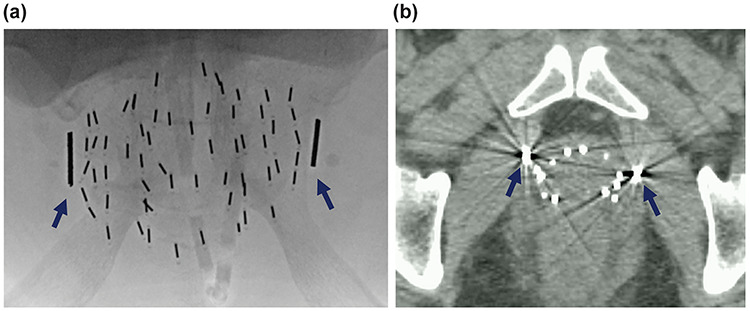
Pelvic radiography (a) and axial computed tomography scan (b) showing the implantation of gold markers (thick arrows) in the surrounding tissue of the prostate.
